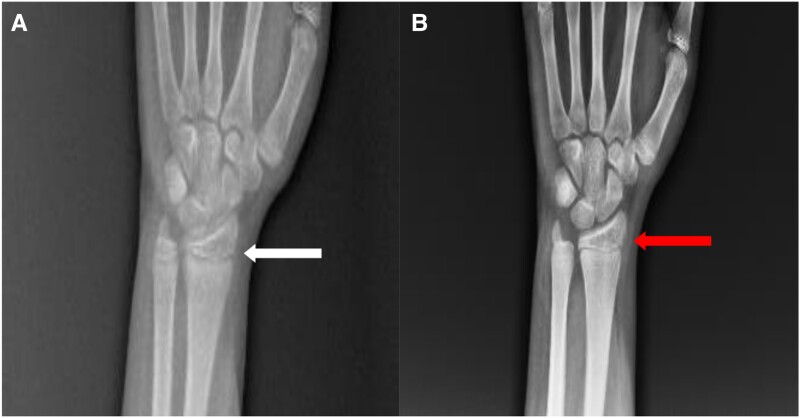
X-rays of left hand wrist joint (anteroposterior view) before (A) and after (B) 6 months of treatment. (A) Metaphyseal fraying and splaying of the distal radial and ulnar metaphyseal (arrow). (B) Resolution of the metaphyseal changes and improvement in deformity after 6 months of therapy (arrow).
